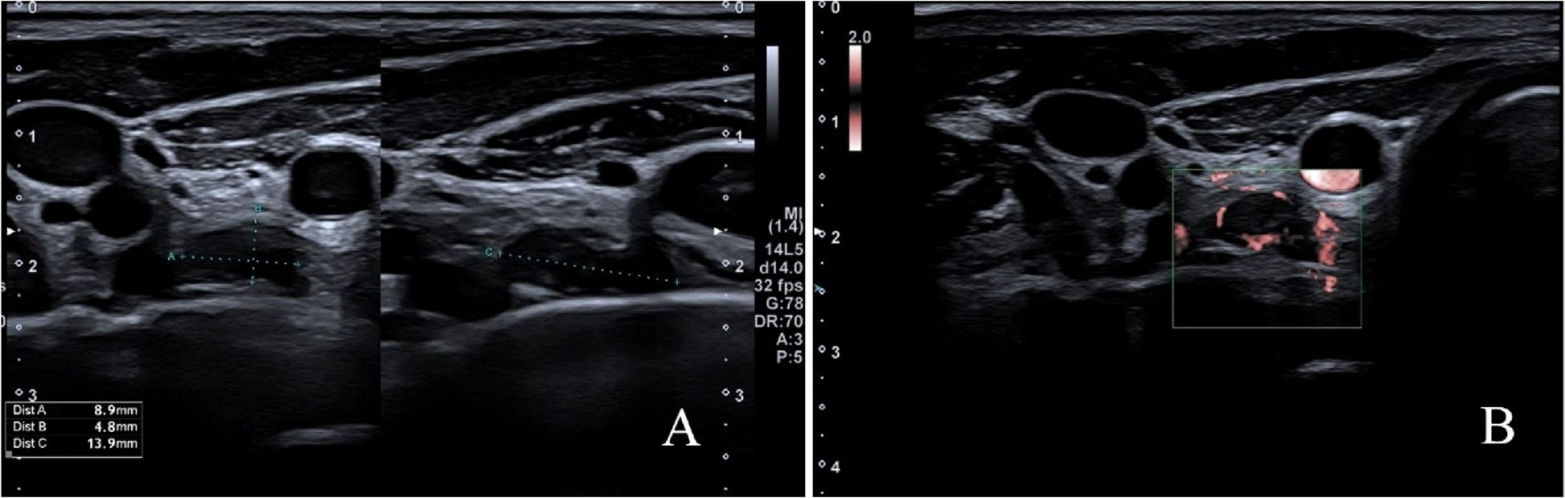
Ultrasound of the neck after thyroidectomy and radioiodine therapy. In postoperative bed presence of previously undetectable mass of hypoechogenic glandular tissue (with increased vascularity) – suspicious of DTC recurrence. (A) Transverse/sagittal section (B)Transverse section – CDI presentation
